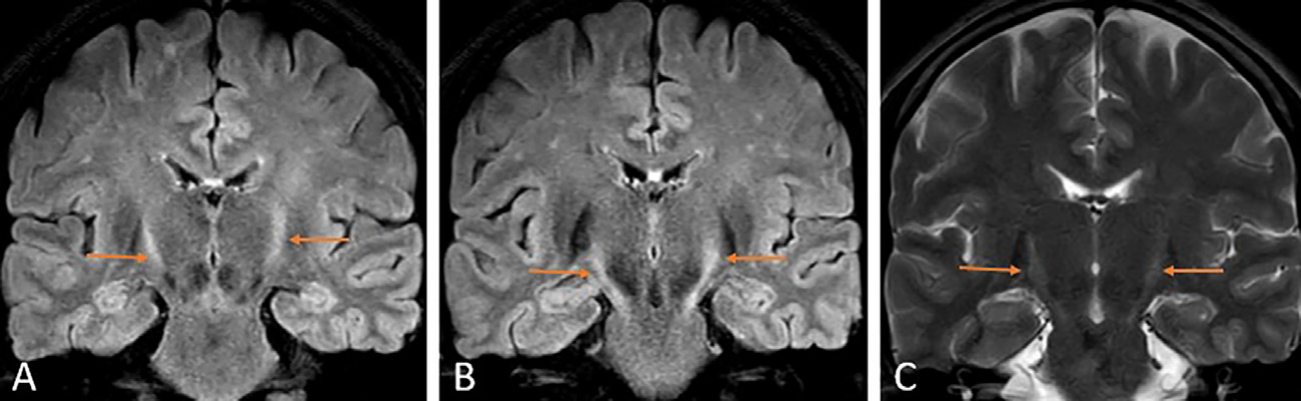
Magnetic Resonance Imaging of the brain coronal sections fluid attenuation recovery sequence (A, B), and T2 weighted imaging (C) showing hyperintense signal involving bilateral corticospinal tracts, bilateral internal capsule, extending superiorly up to the motor cortex and inferiorly up to the cerebral peduncles of the midbrain showing wine glass sign (orange arrows) suggesting amyotrophic lateral sclerosis.
